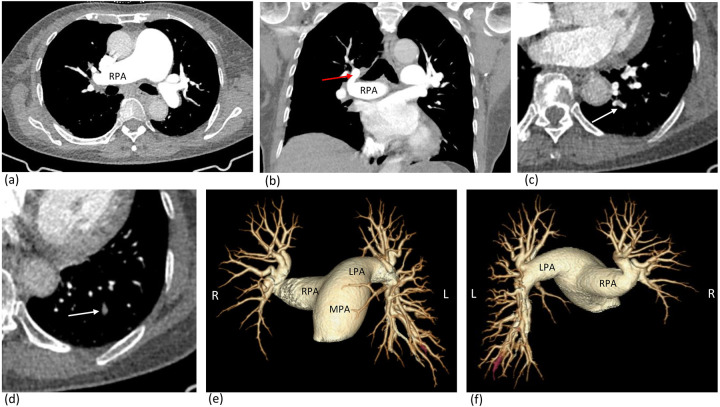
Axial images from contrast-enhanced CT scans (a,b,c,d) and 3D reconstruction (e,f) shows interruption of the pulmonary artery in the right middle and lower lobes, with dilation of the pulmonary artery trunk and left pulmonary artery and pulmonary embolism on distal branch of the left lower lobe pulmonary arteries. Pulmonary embolism indicated by white arrow (c,d) and red highlight (e,f). Pulmonary artery in the right upper lobes indicated by red arrow. LPA left pulmonary artery; RPA right pulmonary artery; MPA main pulmonary artery.
